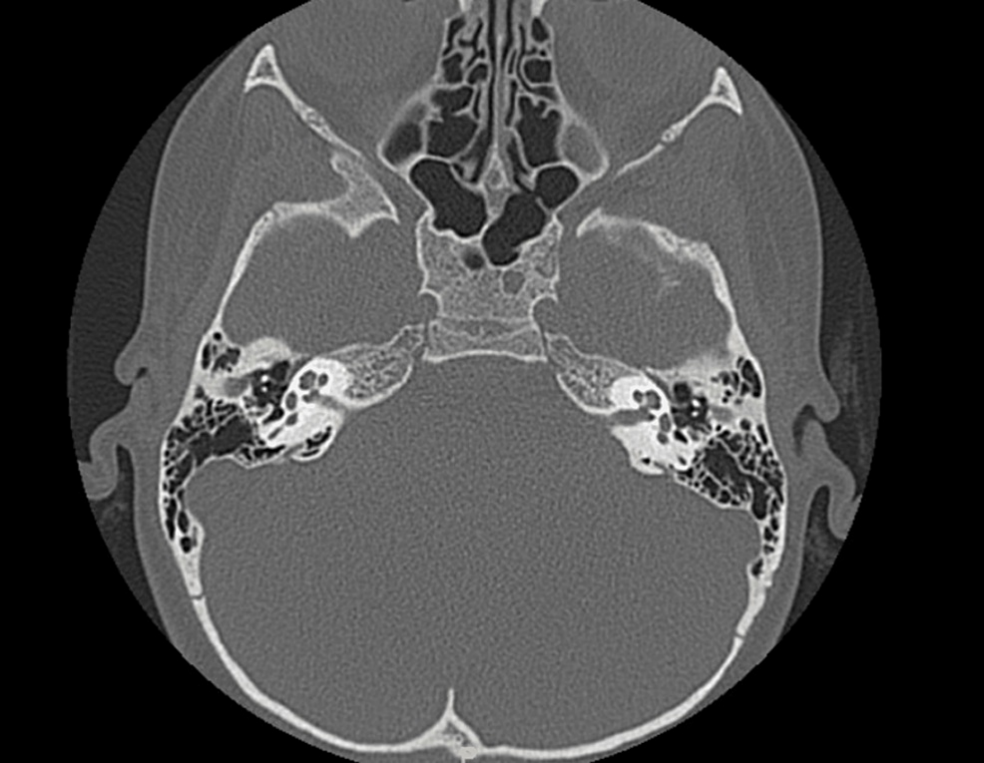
High resolution axial CT IAC without contrast shows bilateral middle ears without abnormalities. IAC: Internal auditory canal.
